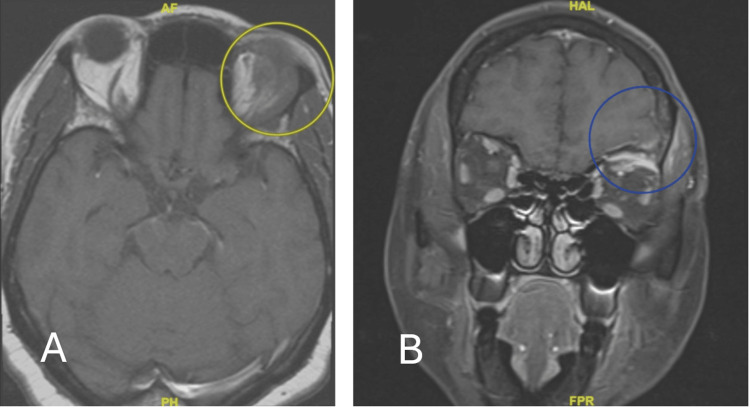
MRI orbits without contrast (March 2023) Abnormal appearance of the superior lateral extraconal soft tissues of the left orbit as denoted by the yellow circle in image A (axial view) and the blue circle in image B (coronal view). Mild superior periorbital soft tissue swelling with mild involvement of the left lacrimal gland is also present. Extraocular muscles, globe, and intraconal soft tissues are normal in appearance.
